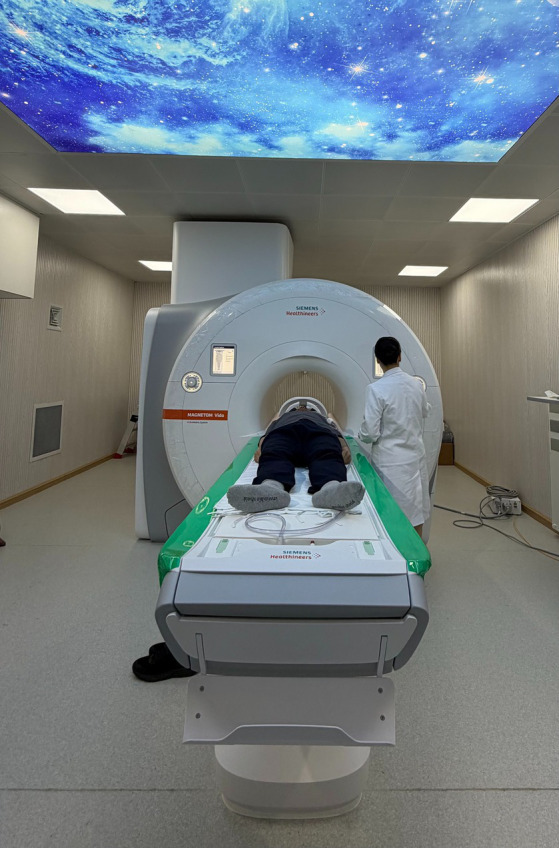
The 3.0 T MRI scanner (PRISMA, Siemens) used in this study.
